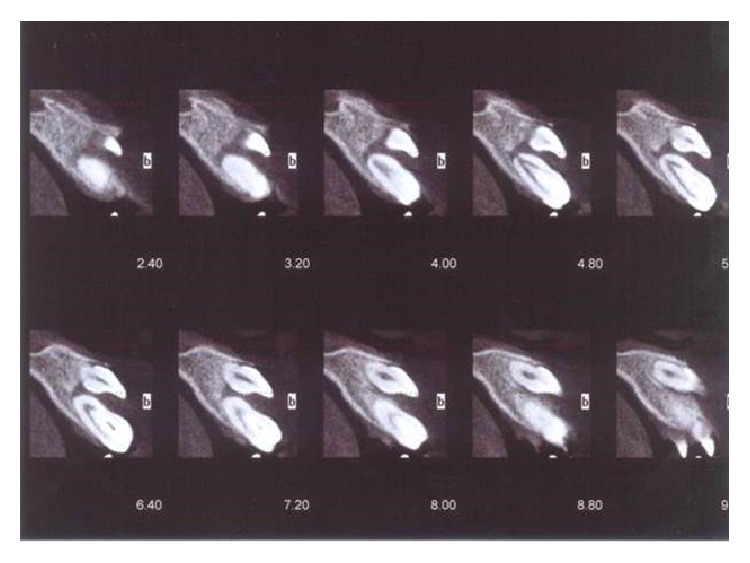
Cone beam computer imaging (CBCT) of lateral view depicting dilated odontoma.
